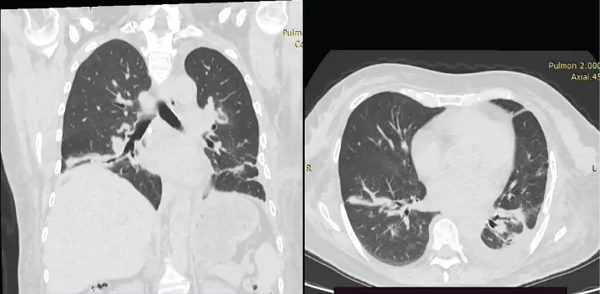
Postoperative chest CT scan showing no residual pleural masses after complete tumor resection.
